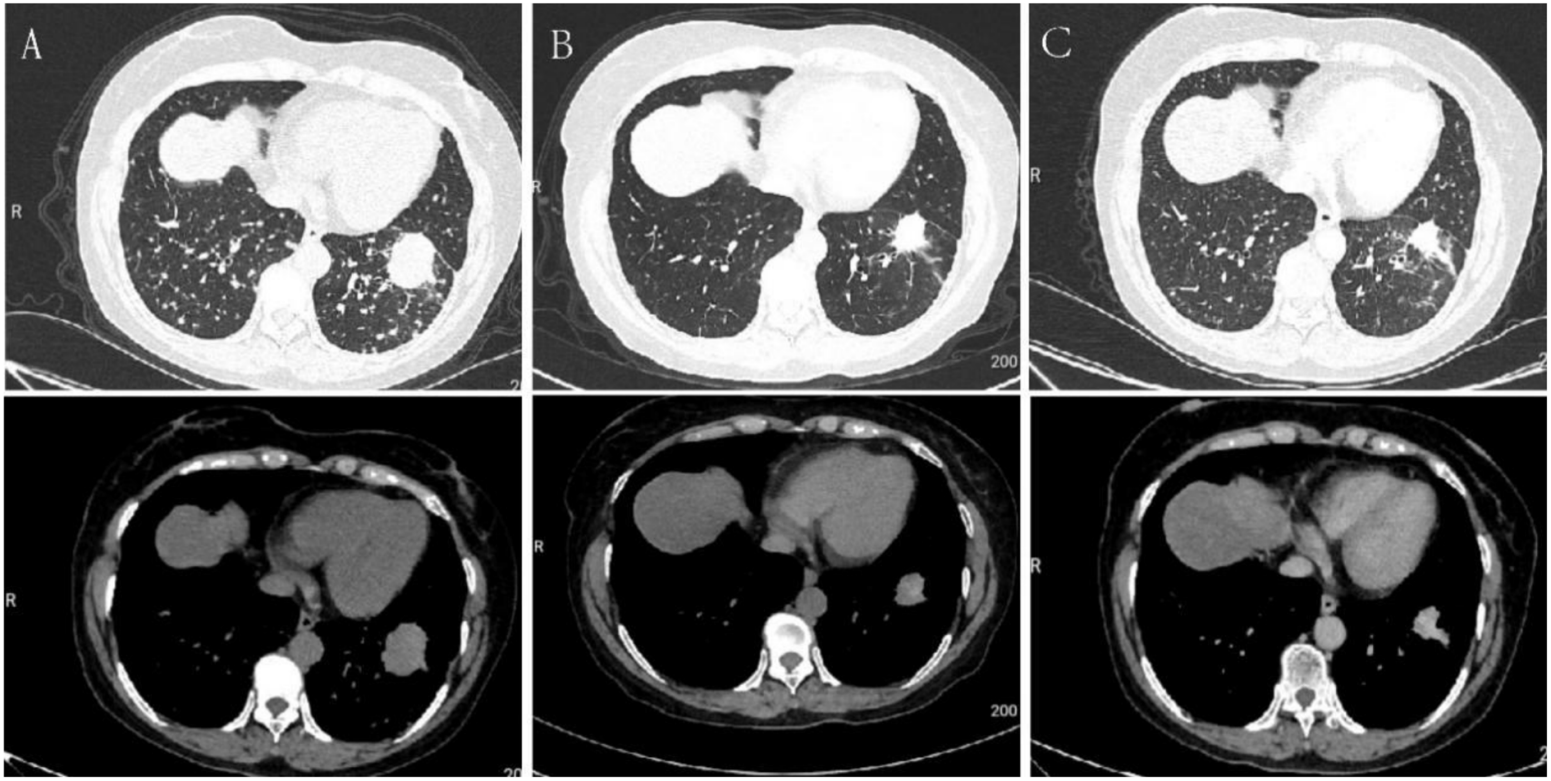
Serial chest CT scans of Case 1 during diagnosis and treatment. (A) Baseline chest CT (December 22, 2023) showing a mass-like lesion in the left lower lobe. (B) After 2 months of treatment (February 2024), the lesion was reduced to 2.8 × 2.5 cm. (C) After 4 months of treatment (April 2024), further reduction of the lesion to 2.7 × 2.2 cm was observed.
